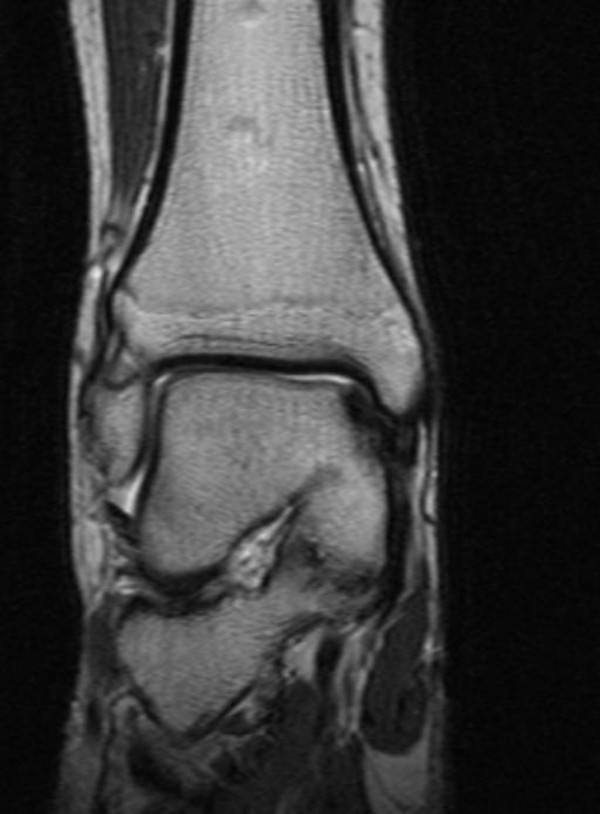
3D coronal CT of the ankle and hid foot showed significant talocalcaneal coalition.
